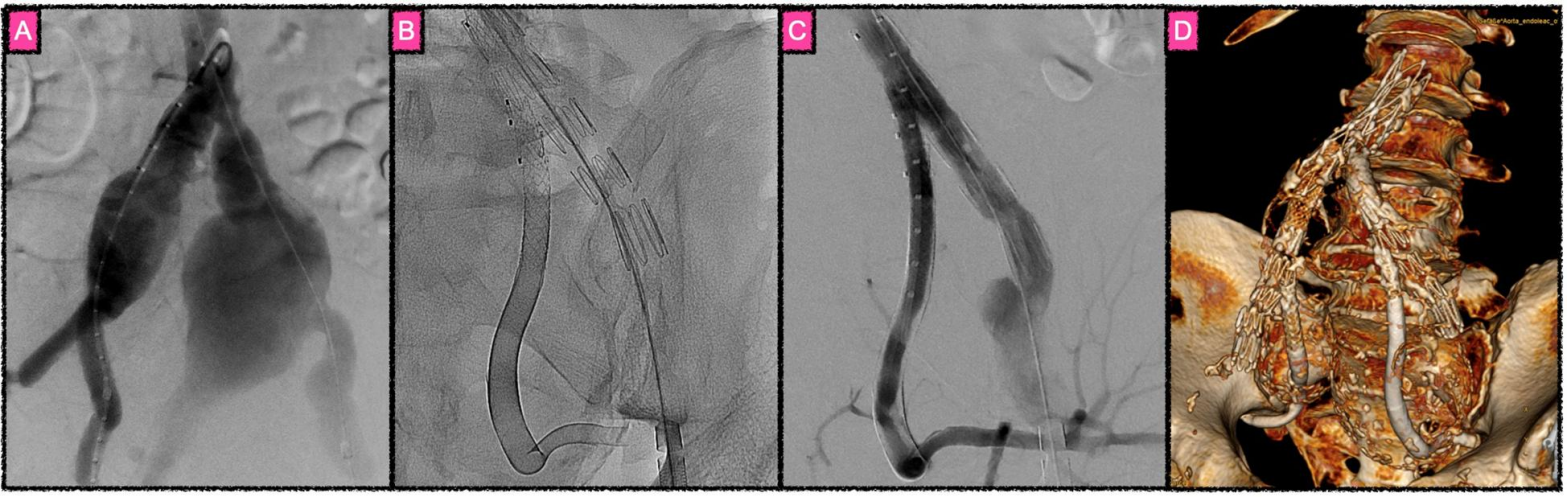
Complex reconstruction of a giant fusiform aneurysm involving the common iliac artery bifurcation and especially the external iliac artery, focusing on long-distance flow diversion for reconstruction of the external iliac artery. (A) Angiographic overview of the aortic bifurcation, both common, external and internal iliac arteries in right anterior oblique projection. At first, the left common iliac artery bifurcation was reconstructed performing T-stent grafting in order to generate a suitable proximal landing zone for the intended FDS construct. Then, the left internal iliac artery was antegradely microcatheterized until reaching the healthy vessel segment distal to the aneurysm. Subsequently, the first FDS was implanted in the distal healthy segment to create a stable anchoring point for the following FDS telescoping construct. Thereafter, five additional FDS were implanted in an overlapping manner from distal to proximal. Given the fusiform nature of the aneurysm, each FDS foreshortened maximally, as no wall attachment was achievable in the aneurysmatic vessel. The last, most proximal FDS was landed within the T-branch of the stent graft construct. The FDS were implanted with a minimum of 30% material overlap, so that sufficient stability of the telescoping construct was guaranteed. (B) Radiographic image of the FDS implants in the left-hand side internal iliac artery. A total of six FDS cover the entire aneurysm with a secure distal landing zone in the healthy segment and a secure proximal landing zone in the T-branch of the stent graft construct. (C) Subtracted DSA image after reconstruction. Besides the common and external iliac artery, only the lumen within the FDS construct in the internal iliac artery is opacified; the aneurysm outside the stents is already occluded by thrombus. Despite the high density of the braid mesh created by the overlapping FDS, covered side branches of the internal iliac artery remain sufficiently perfused in a timely normal fashion. (D) 3D reconstruction of a computed tomography performed 3 months after reconstructing the contralateral fusiform aneurysm in a similar manner.
